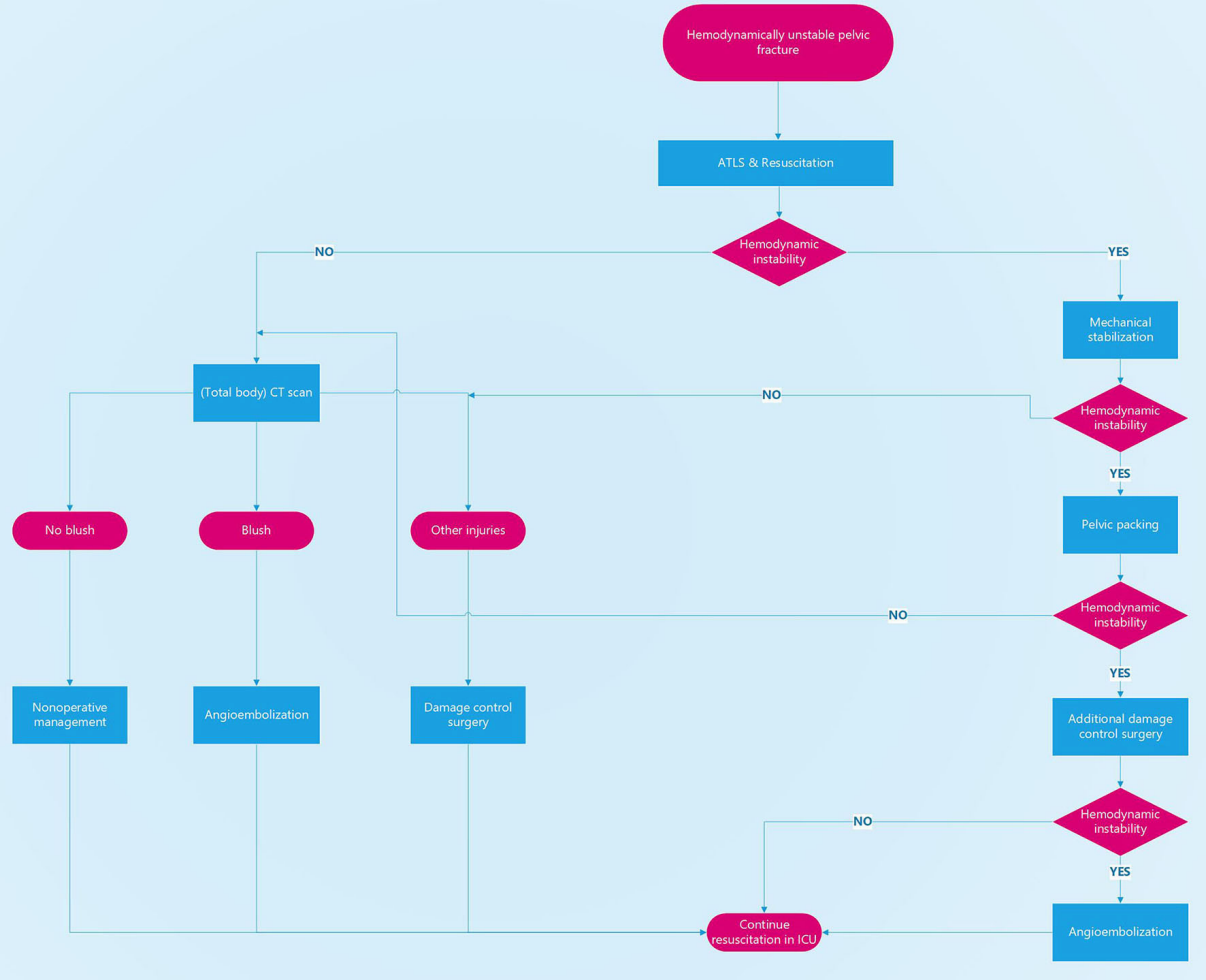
Treatment algorithm for hemodynamically unstable pelvic fractures. Decision-making is based on the patient's physiological status. Hemodynamically unstable patients should not be taken to a CT scanner or to the angio suite but to the operating theater for mechanical stabilization, pelvic packing, and additional damage control surgery if needed.
